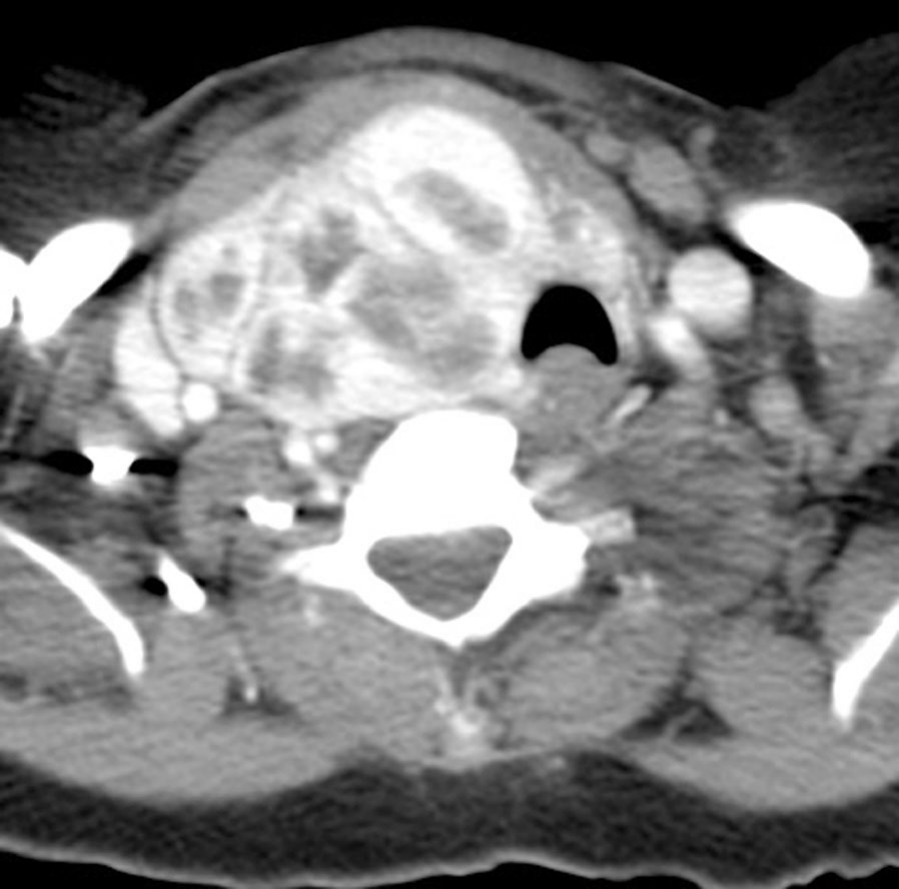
Axial contrast enhanced CT at the level of the thyroid gland. Left thyroidectomy noted with multinodular enlargement of the right thyroid lobe with marked tracheal deviation to the *left*
